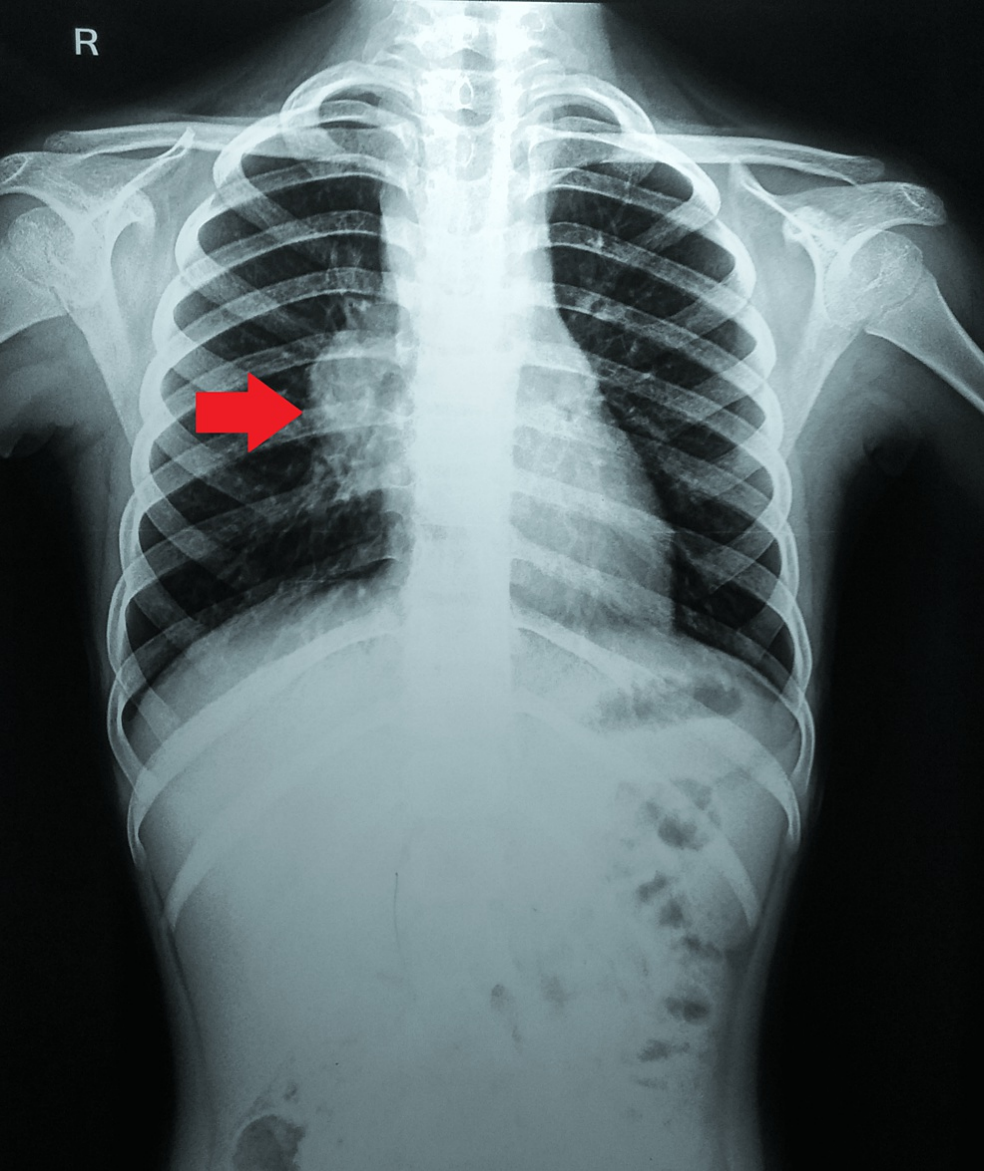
Plain chest radiograph posteroanterior view Arrow showing right hilar lymphadenopathy
